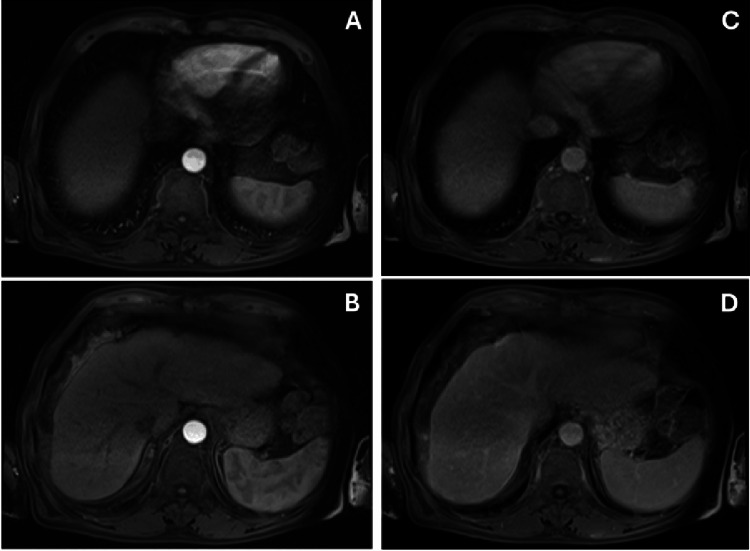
T1-weighted abdominal MRI images, performed six months after excision of hepatic nodules, with no visible focal lesions, showing no recurrence of hepatocellular carcinoma. A, B: arterial phase; C, D: venous phase
